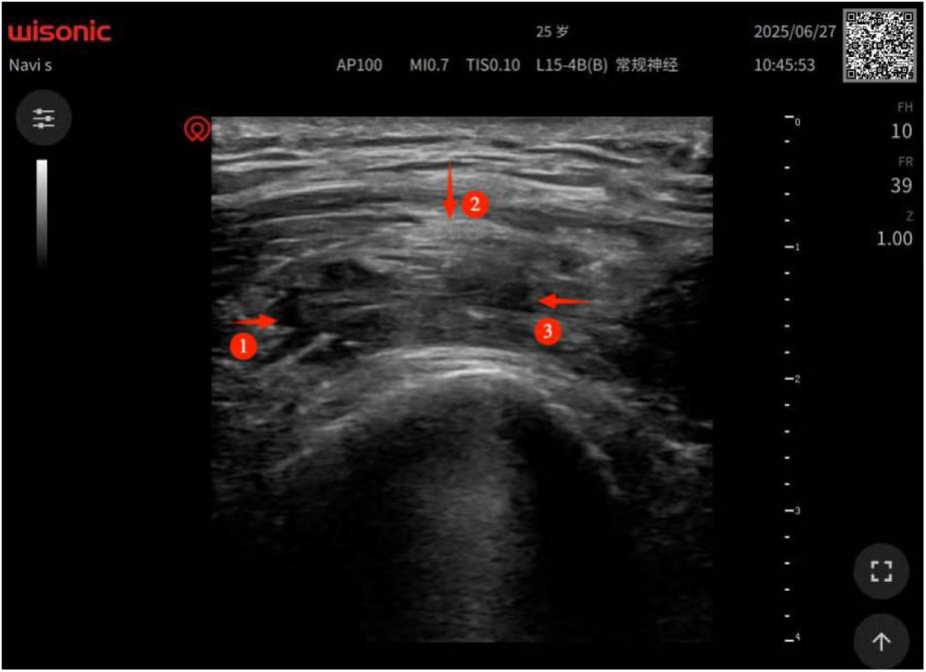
Preoperative ultrasound image: three main adhesive regions (indicated by three red arrows): ① adhesion at the attachment site of the patellar tendon to the tibial tuberosity; ② adhesion between the quadriceps tendon and the superior pole of the patella, showing uneven echo and poor mobility; ③ synovial-capsular adhesion in the medial tibiofemoral joint space accompanied by a sonolucent area, which is considered to be associated with old bleeding.
